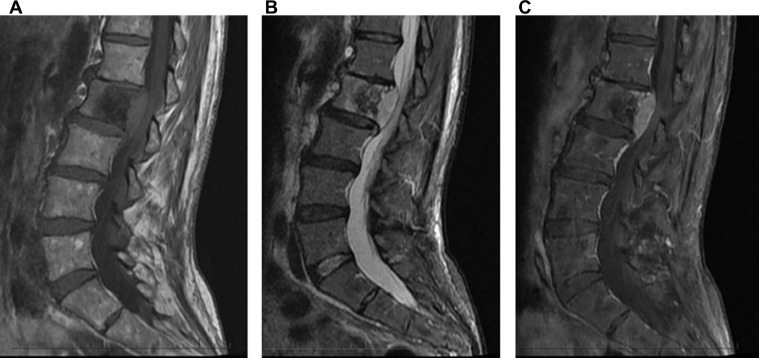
MRI of the lumbar spine including (A) T1, (B) STIR, and (C) postcontrast T1 fat-saturated sagittal images demonstrate a T1 hypointense and STIR heterogeneous lesion predominantly in the posterior aspect of the L1 vertebral body, extending from superior to inferior endplate and with irregularity of the posterior cortex. The anterior aspect of the vertebral body is spared. There is an associated epidural soft tissue component along the posterior aspect of the L2 vertebral body which is T1 isointense (relative to disk) and STIR hyperintense with homogenous enhancement; this results in spinal canal stenosis with compression of the thecal sac and conus medullaris/cauda equina at this level. There is heterogeneous marrow signal in the other vertebrae without a focal lesion. Degenerative disc disease is noted at L1-L2. The conus medullaris terminated at the mid-L2 level in this patient.
